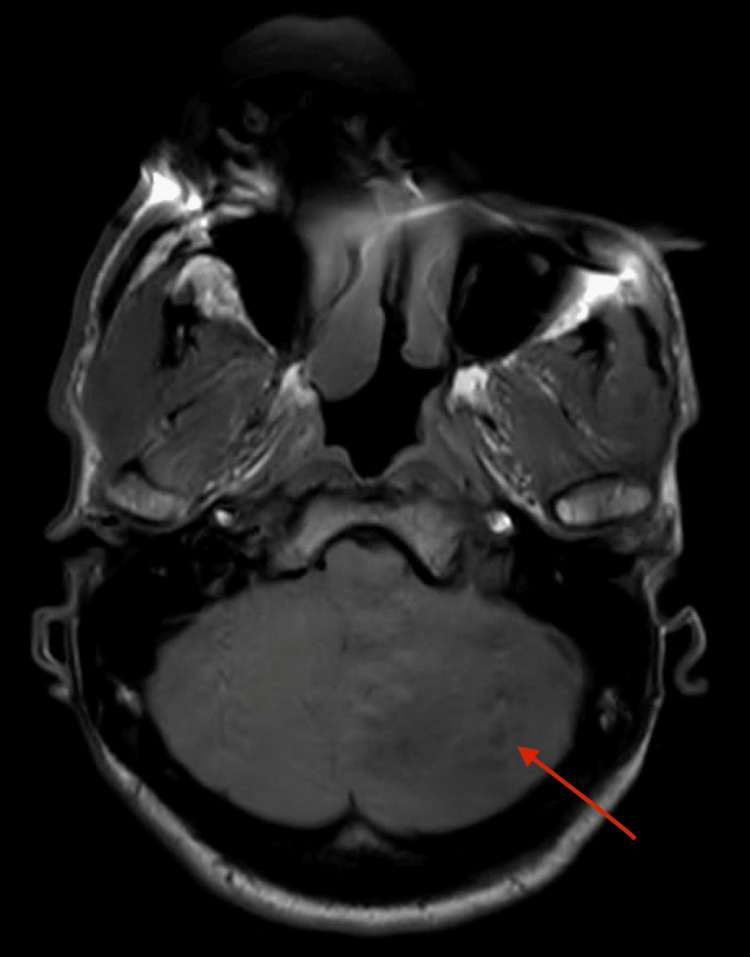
T1 series of magnetic resonance imaging (MRI)
